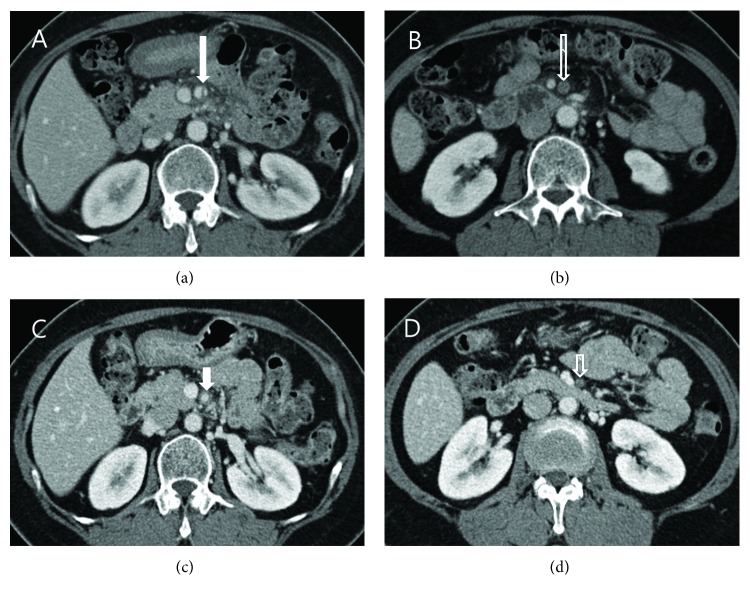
Computed tomographic angiography (CTA) findings in a 55-year-old woman with spontaneous isolated dissection of the superior mesenteric artery (SMA) at the initial presentation and at the 9-month follow-up exam. (a, b) Axial images show the dissection flap (long arrow) in the main trunk of the SMA and complete obstruction of the SMA, due to thrombosis (long empty arrow), at the initial presentation. (c, d) Axial CTA images at the 9-month follow-up exam show the SMA with a patent lumen at the proximal portion (short arrow) and a completely obstructed lumen at the midportion (short empty arrow).
